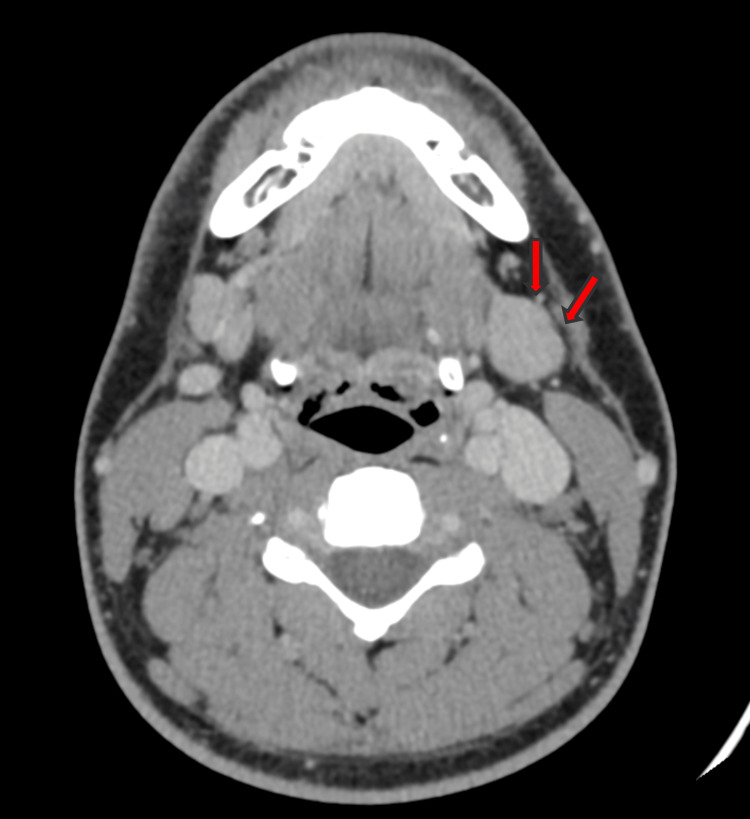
Axial CT image showing right submandibular cervical lymph node with an irregular contour suggestive of a suspicious lesion (arrows)
